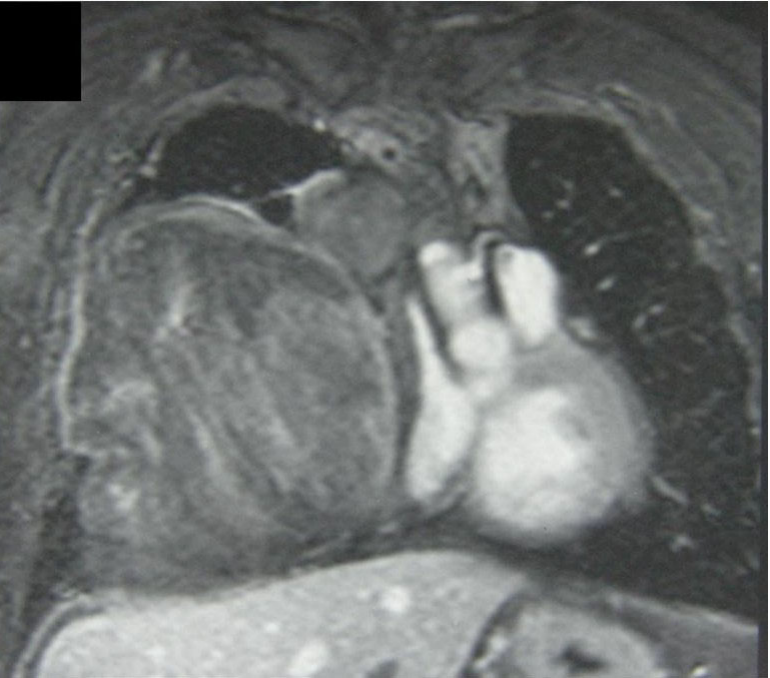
MRI sagital scan showing mass extending into the mediastinum and right pleural cavity. This scan shows clearly the anatomical relationship between the tumour and one of the vessels of the aorto-coronary by-pass.
